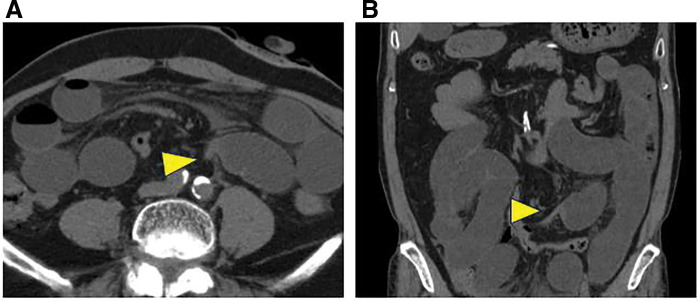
Preoperative CT imaging. Preoperative CT revealed a caliber change in bowel dilatation in the left middle abdomen (A, axial section; B, coronal). No significant signs of strangulation were identified, and the postoperative diagnosis was mechanical small-bowel obstruction. The arrowheads indicate the caliber change at the transition point of obstruction.
